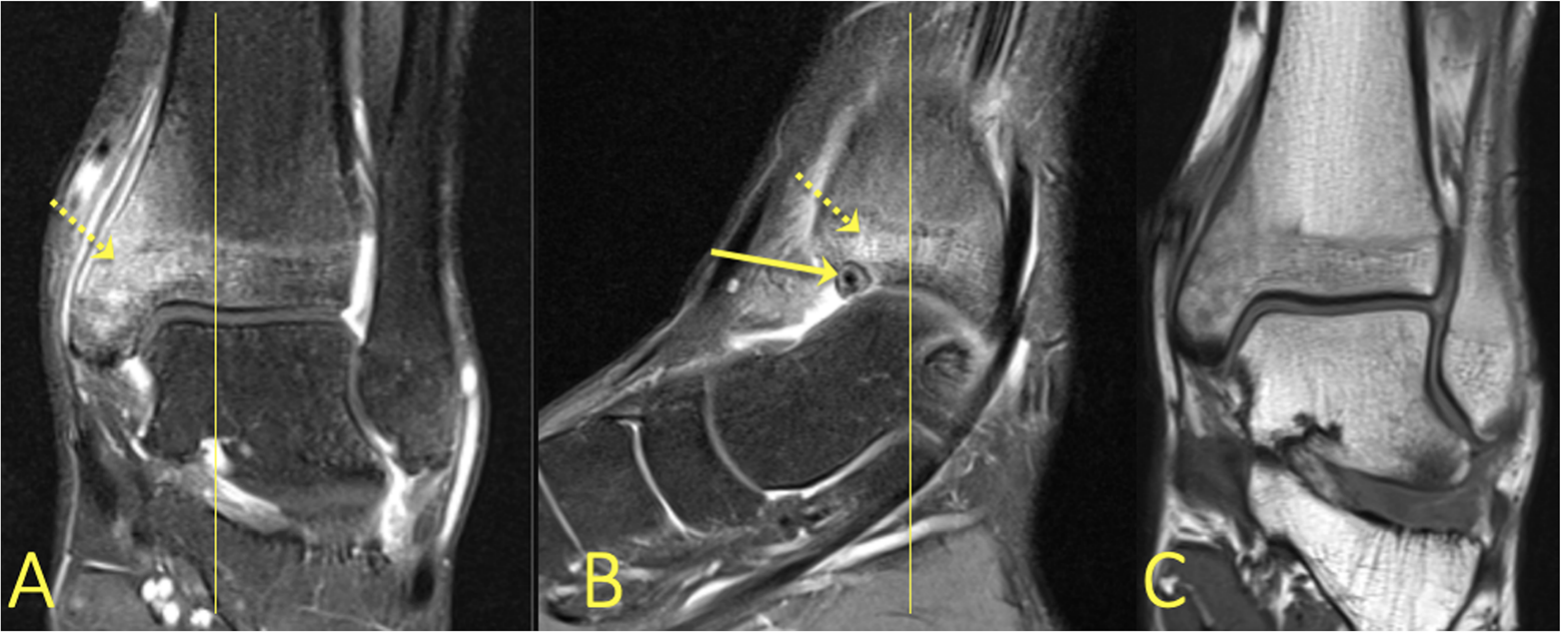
A 19-year-old male presented with nightly ankle pain. MRI confirmed a suspected osteoid osteoma, showing an intra-articular osteoid osteoma (arrow) with minimal sclerosis and extensive BME (dashed arrows) as a perilesional reaction
